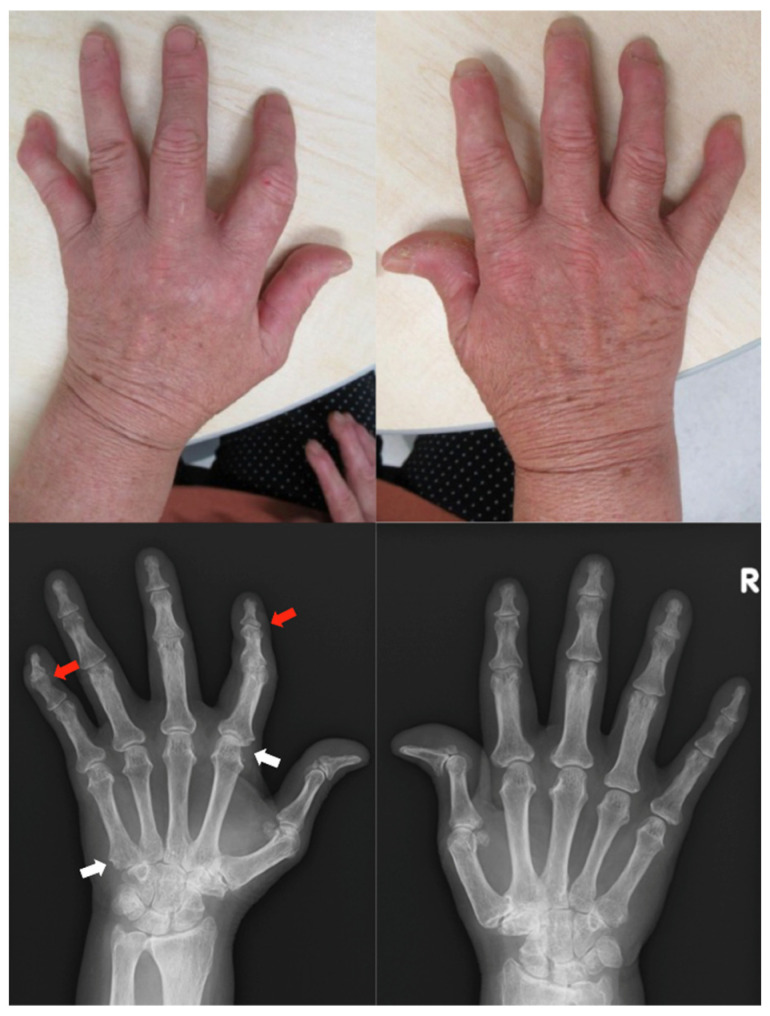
Clinical photographs and radiographs of the hands in case 3. Swan-neck deformities of the left fifth finger and both hitchhiker’s thumbs are shown. Radiographs depict joint space narrowing in the left second and fifth DIP joints (red arrow). Radiographs show subluxation of the left second MCP joints and bone erosions at the left fourth PIP and wrist joint (white arrow). MCP, metacarpophalangeal; PIP, peripheral interphalangeal; DIP, distal interphalangeal.
